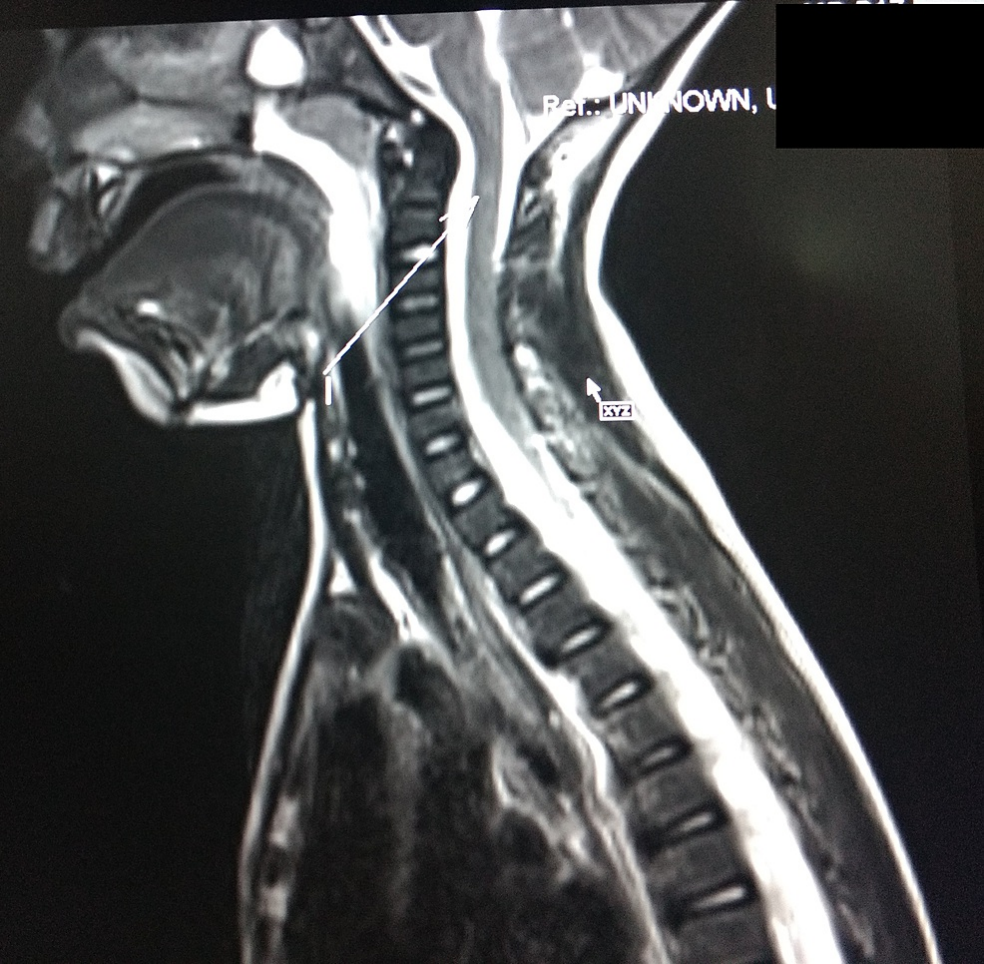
Saggital section MRI spine showing intramedullary T2W/TIRM hyperintensity in the cervical cord, notably in the upper cord at C2, C3, and upper C4 levels MRI: magnetic resonance imaging
